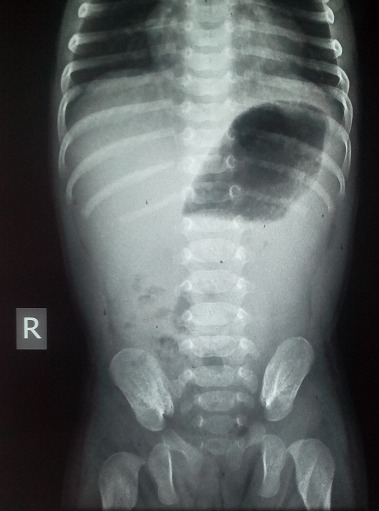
Plain abdominal X-Ray (erect) showing single huge air bubble in the stomach
